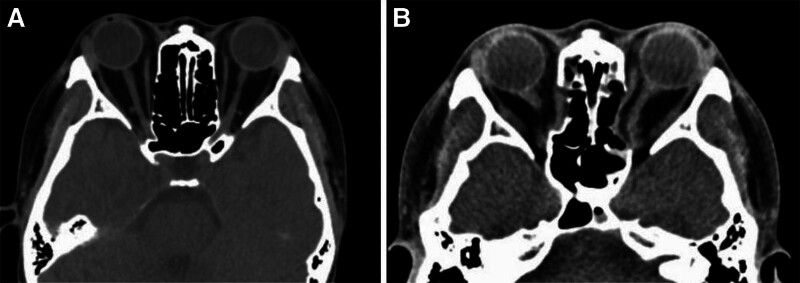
The results of preoperative and postoperative orbital CT. Preoperative orbital CT showed the left eye was obviously convex and the right extraneous eye decreased (A). Postoperative orbital CT showed the changes after bilateral orbital decompression (B). CT = computerized tomography.
